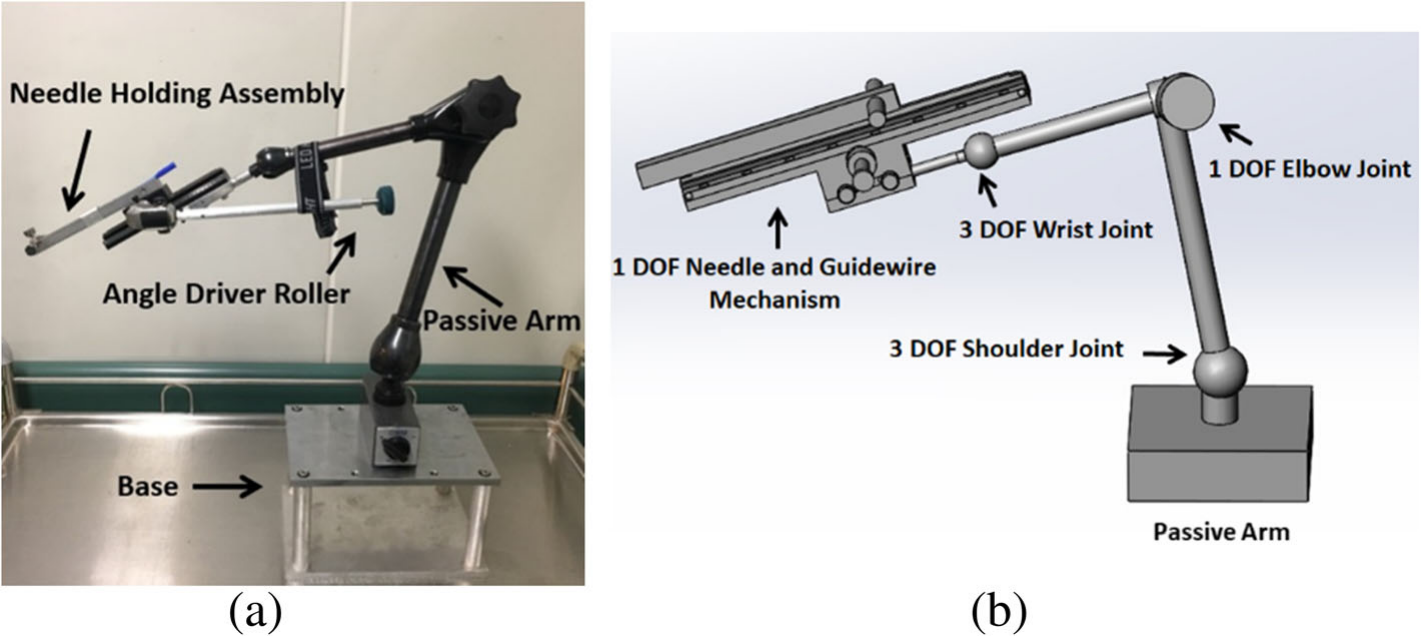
Passive arm and needle holding assembly. a Seven-degree of freedom (DoF) passive arm with 1-DoF needle holding assembly. Long angle driver roller allows the physician to perform arterial puncturing away from the x-ray source. Base could be easily fixed on the patient bed. b Three-dimensional model of the passive arm and needle holding assembly
